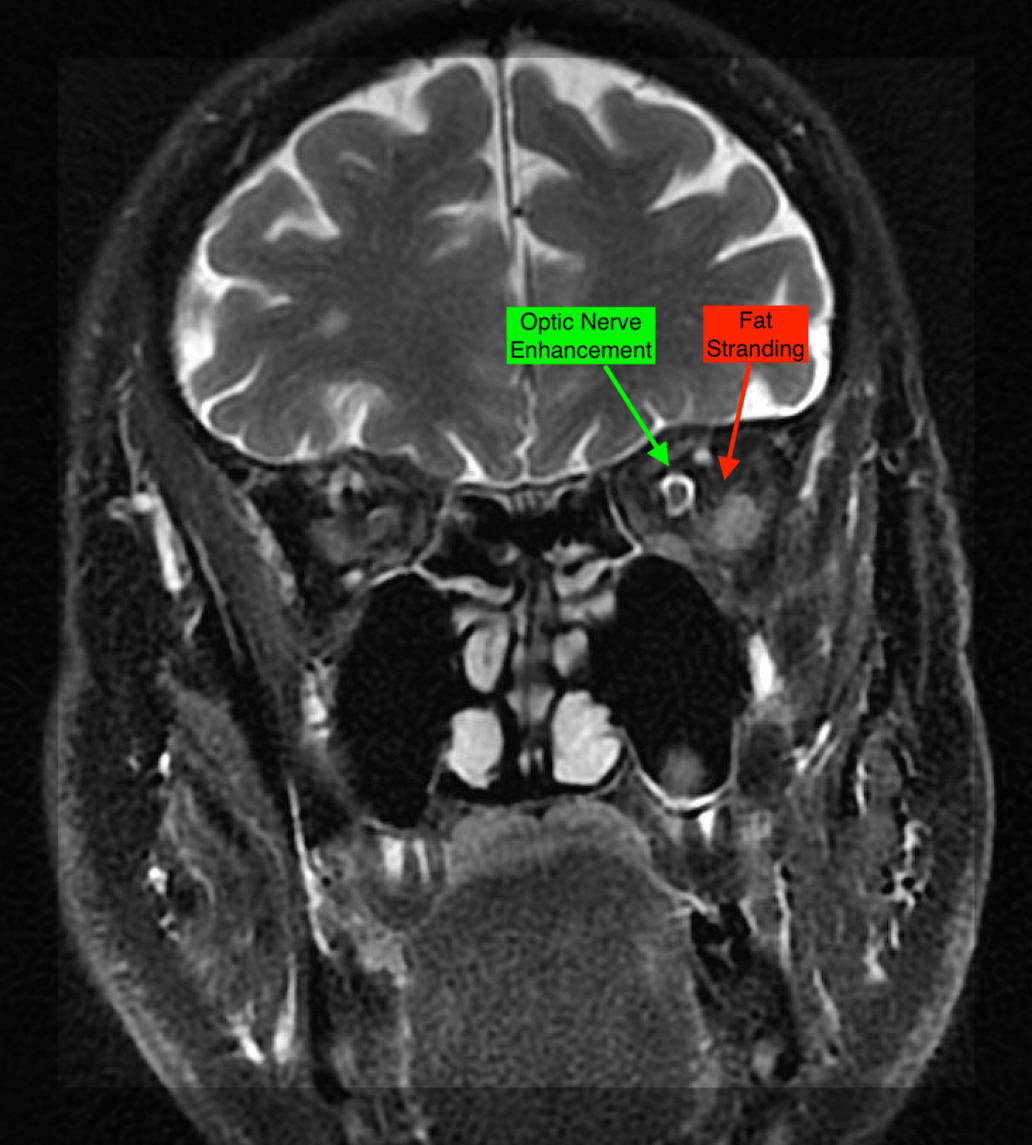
T2 fat-suppressed MRI image depicting left eye fat stranding and increased optic nerve sheath signal
